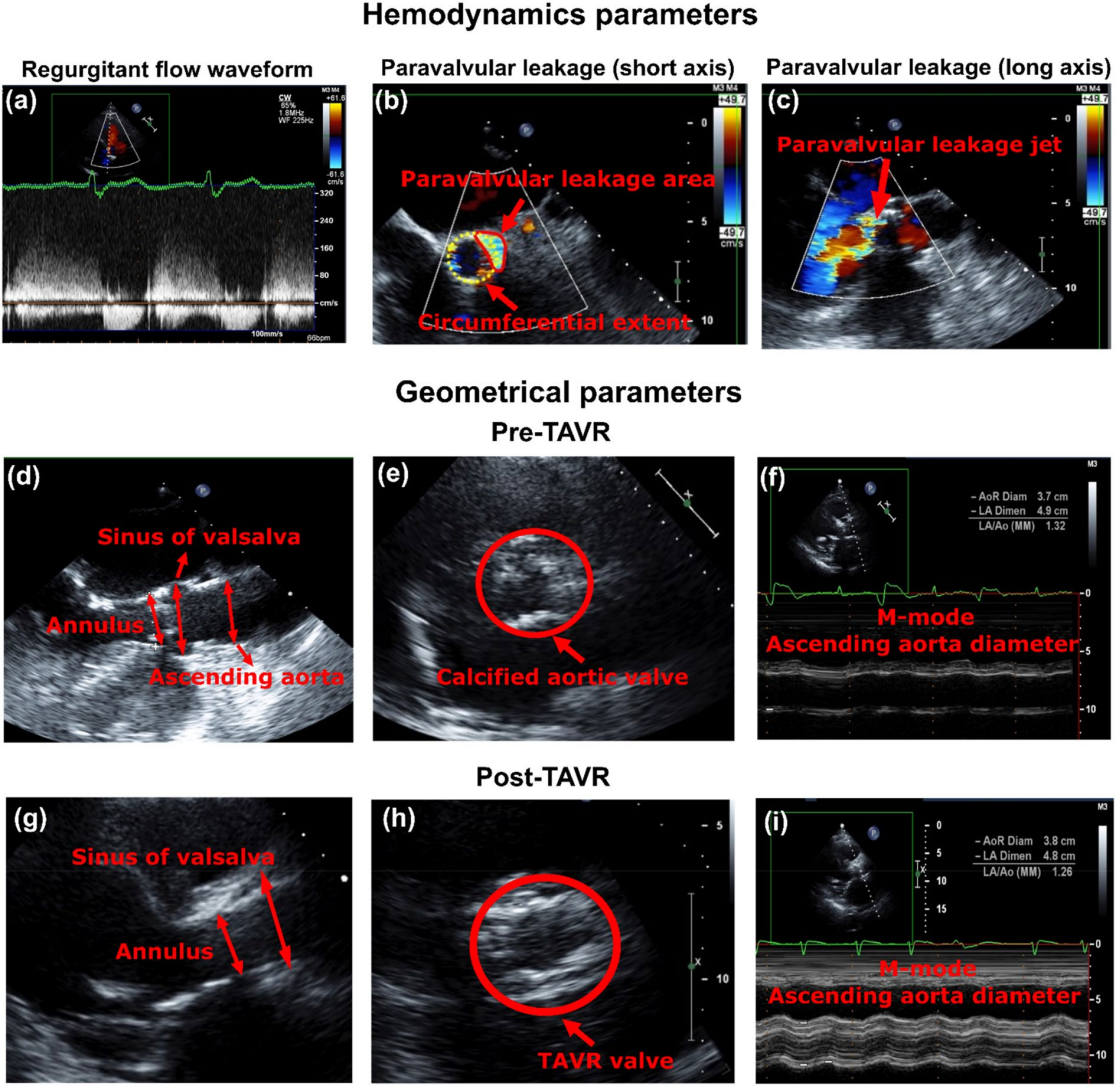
Hemodynamics parameter: (a) Regurgitant flow velocity waveform at the paravalvular leakage cite (Apical 5 chamber view); (b) Parasternal short-axis color doppler view of the prosthetic valve and the paravalvular leakage area (Vena contracta area: 1.04 cm^2^) and its circumferential extent with respect to prosthetic valve diameter (35%); (c) Long axis color doppler view of paravalvular leakage jet interaction with diastolic flow behind the posterior mitral valve leaflet. Geometrical parameters: (d) Parasternal long-axis view associated with different parts of the aortic root and ascending aorta before TAVR; (e) Parasternal short-axis view of aortic valve before TAVR; (f) M-Mode measurement of ascending aorta before TAVR; (g) Parasternal long-axis view associated with different parts of the aortic root, prosthetic frame and ascending aorta after TAVR; (h) Parasternal short-axis view of TAVR; (i) M-Mode measurement of ascending aorta after TAVR.
